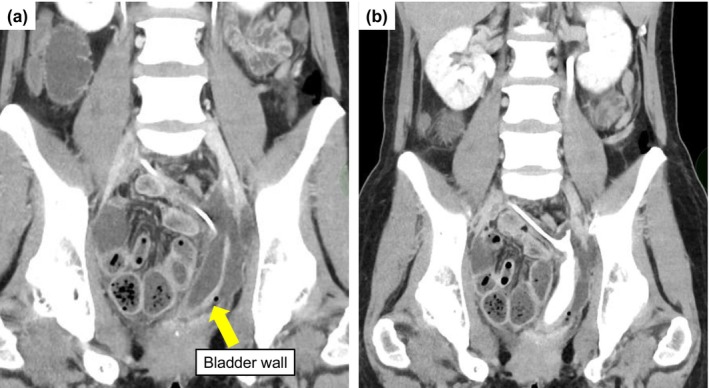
Postoperative contrast‐enhanced CT of the bladder. (a) Early phase demonstrated enhancement of the entire bladder wall. (b) The bladder was deviated to the left side without no obvious urinary leakage was observed.
